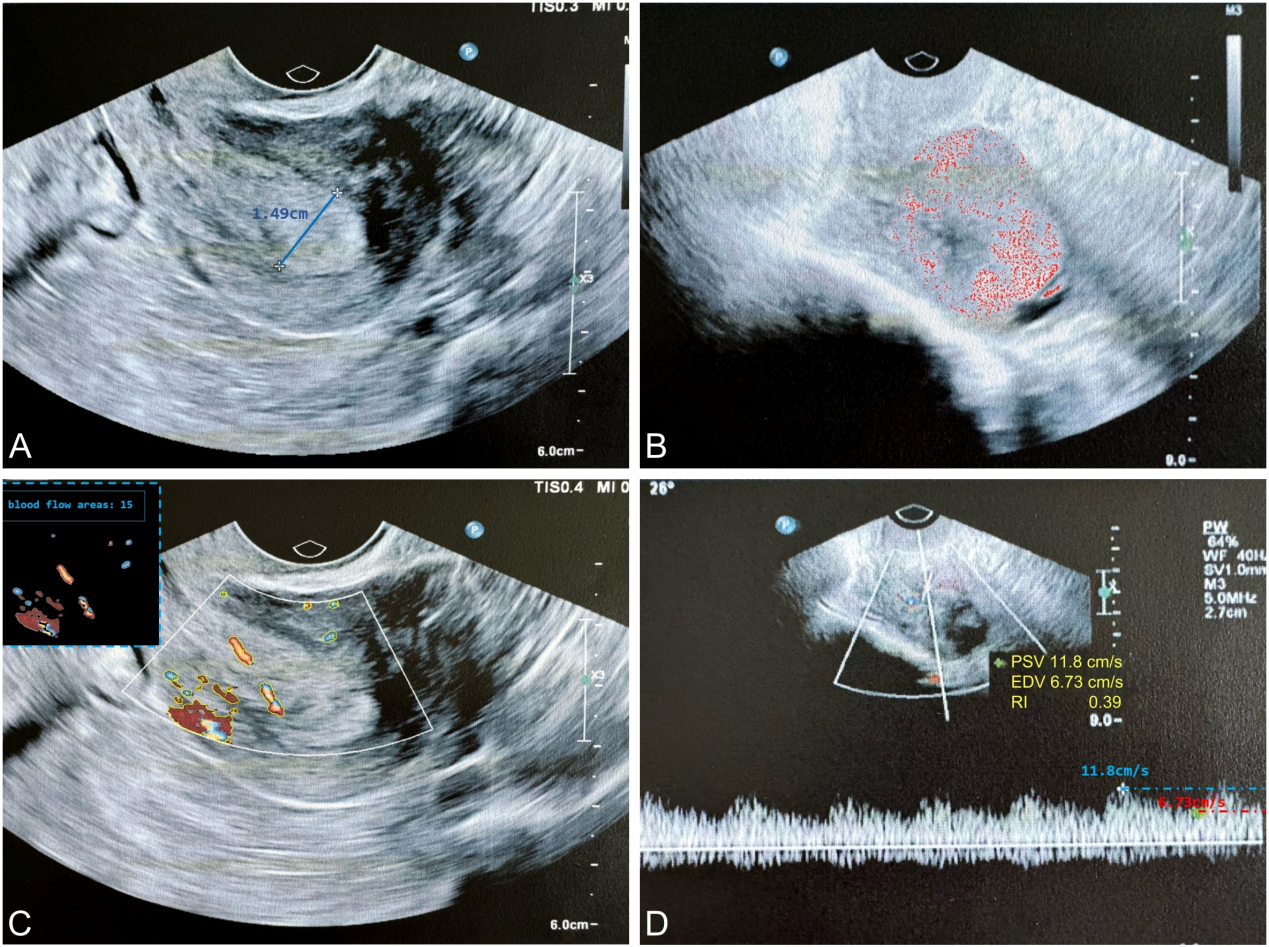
The typical ultrasound exploration image of EC highlights key diagnostic features. (A) The endometrial thickness was measured at 1.49 cm (thickness ≥ 0.5 cm suggests a higher probability of malignancy); (B) Ultrasound examination revealed heterogeneous echogenicity within the tumor, suggesting a higher probability of malignancy; (C) Color Doppler imaging demonstrated increased vascularity within the tumor, characterized by a punctate or arborescent distribution pattern, suggesting a higher probability of malignancy; (D) Spectral Doppler analysis revealed a RI < 0.4, indicative of neoangiogenesis. EC, endometrial cancer; RI, resistance index.
